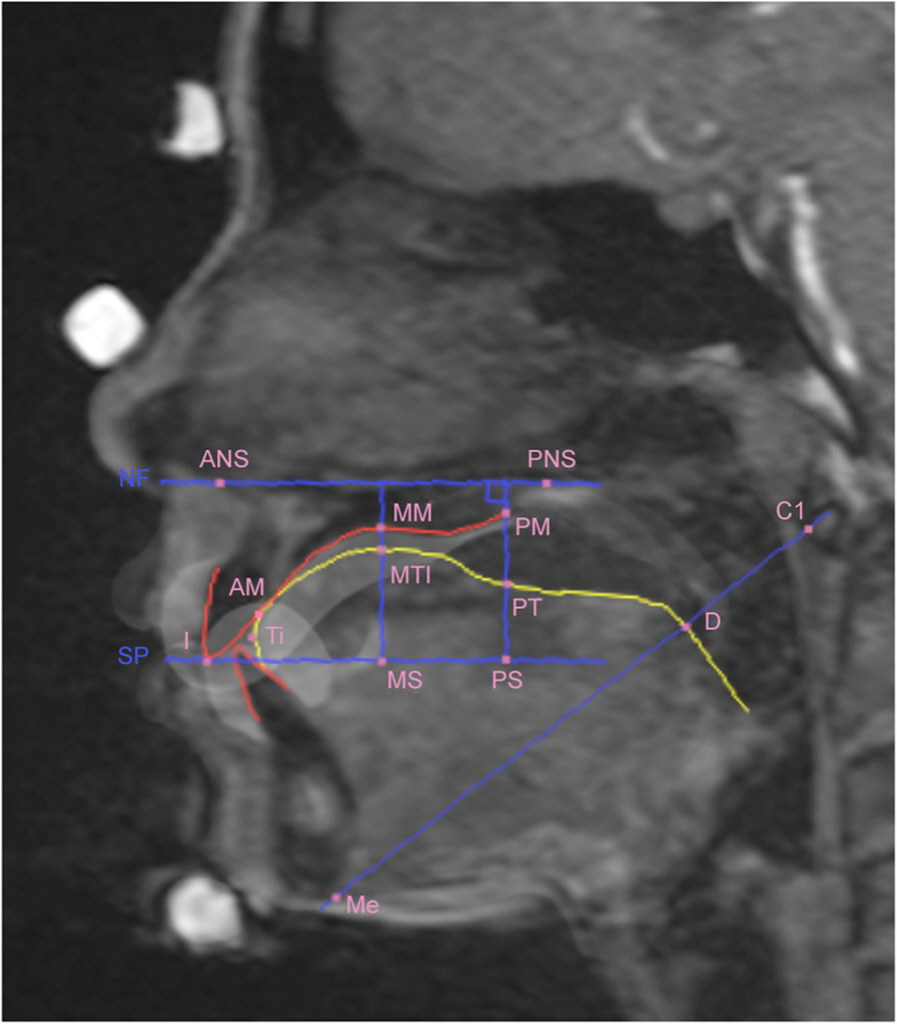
Lines and points on the cine-magnetic resonance image after superimposition of the static image. Red line: tracing of the upper incisor, hard palate, and lower incisor. Yellow line: tracing of the tongue. Blue line: the planes used in the analysis. Pink dots: the points used in the analysis. Please refer to Table 1 for abbreviations
